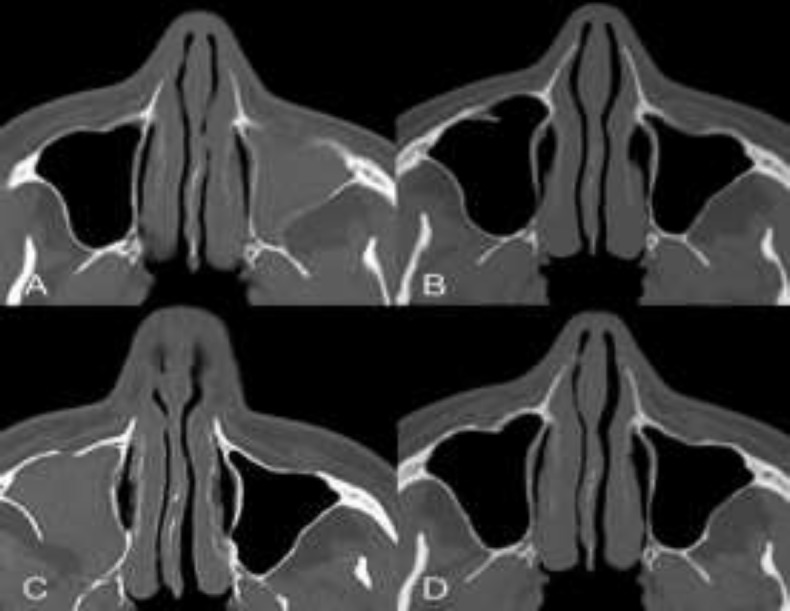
A): Axial computed tomography scan showing pre-operatory left silent sinus syndrome with left retro-antral fat pad expansion and demineralization of the anterior and lateral walls of the maxillary sinus, B) Left maxillary sinus re-ventilated ten months after the first surgical operation, C) Occurrence of right silent sinus syndrome with retroantral fat pad expansion and thinning of the posterior wall after 2 years, D) Post operatory scan 5 years after the second surgical operation
